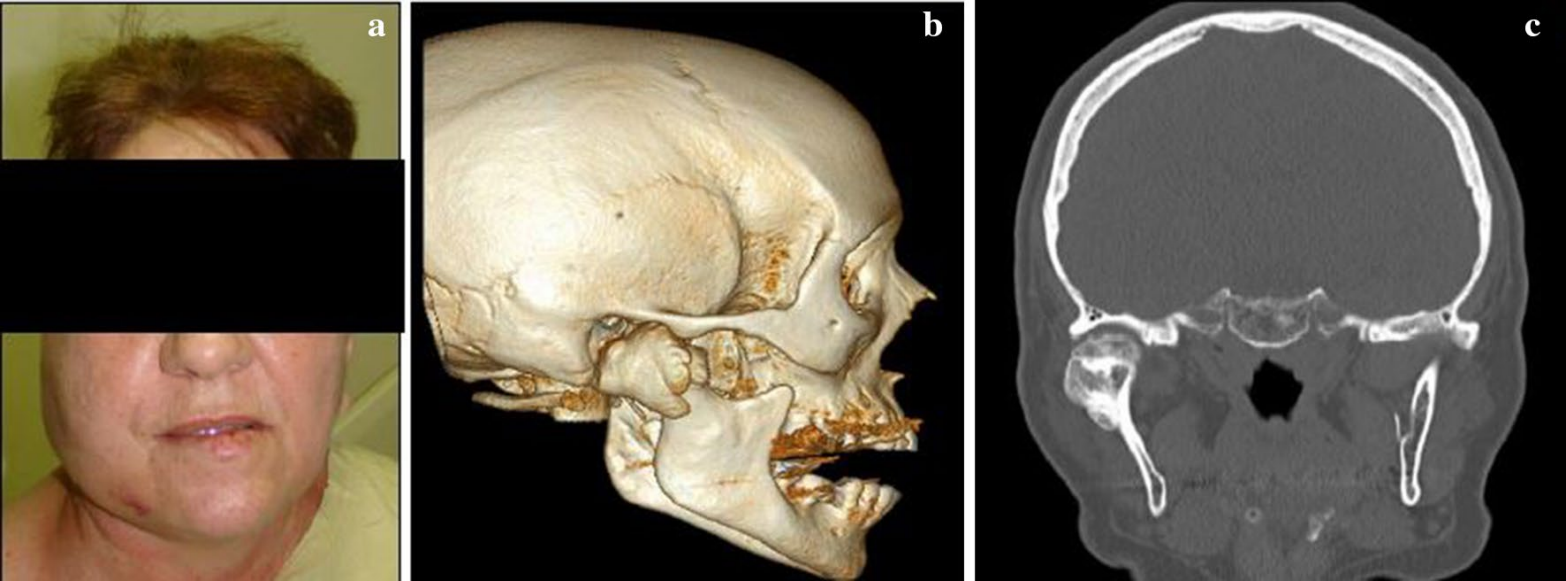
a The asymmetry in the left lower jaw of the patient; b, c 3D CT, note the large tumor on the right condyle, from two different angles
